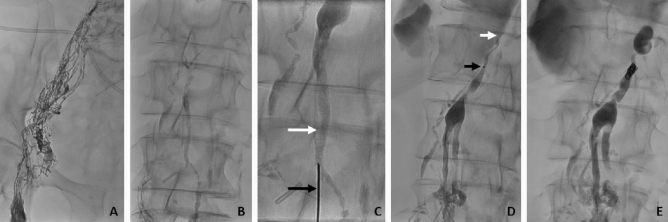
71-year-old woman referred for interventional treatment of bilateral, intractable chylothorax after oesophagectomy due to oesophageal cancer with drainage volumes > 2000 ml/day. X-ray lymphangiography was performed through an inguinal nodal access (A) leading to opacification of retroperitoneal lymphatics (B). (C) Fluroscopically-guided transabdominal puncture of the left lumbar trunk (white arrow) below the cisterna chyli was performed with a fine-needle (black arrow). After successful access, a micro-catheter (catheter tip: short black arrow) was advanced into the thoracic duct over a micro-wire (D) and transcatheter ductography showed transection (short white arrow) of the thoracic duct with extravasation into the thorax. After embolization with micro-coils and liquid embolic (E) the chylothroax resolved completely.
